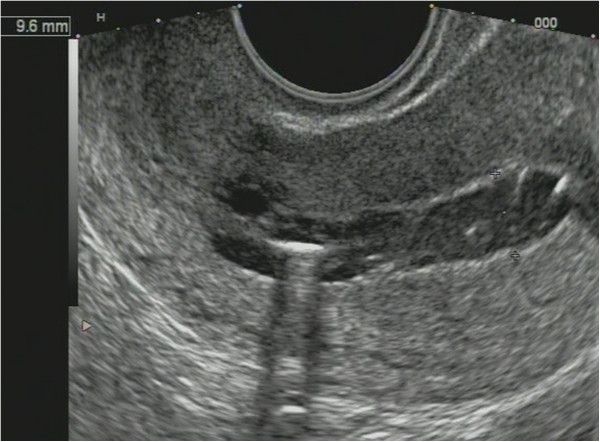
Uterine cavity after hysteroresection. Sonogram of unfavorable outcome.
